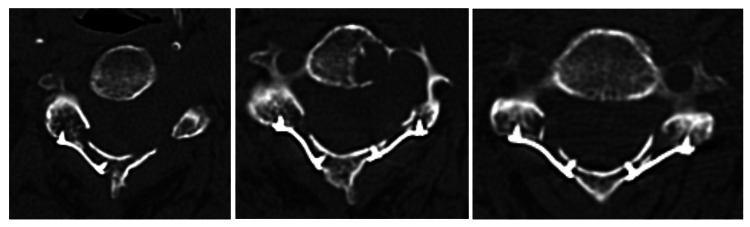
Post-operative CT images of screws at C3 (left), C4 (middle), and C5 (right) levels.
